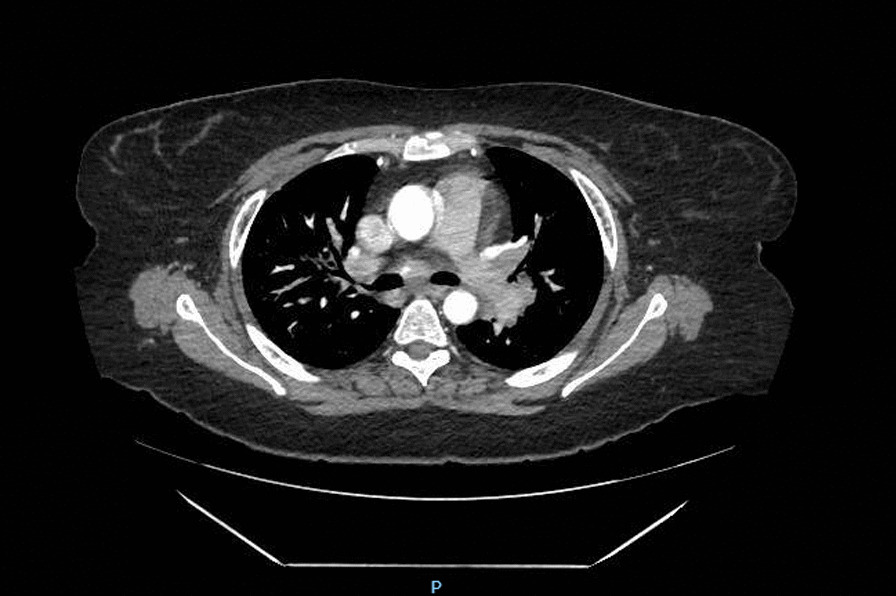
Bilateral hilar adenopathies present in chest CT
